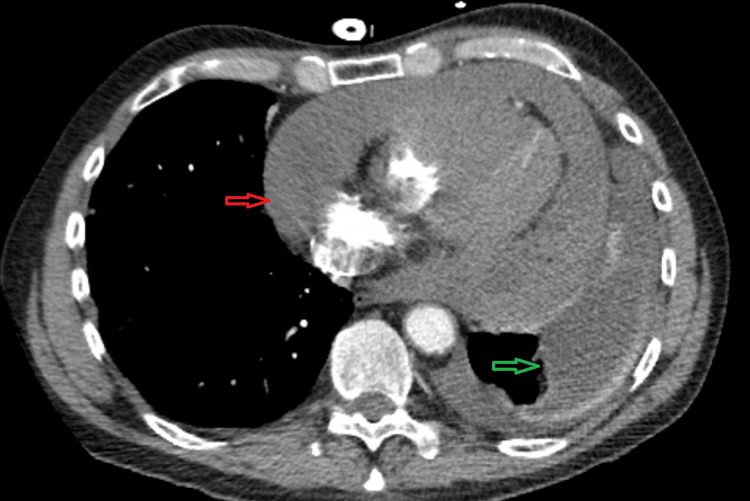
CT angiogram of the chest A large pericardial effusion (red arrow) and left-sided pleural effusion (green arrow) are observed.
